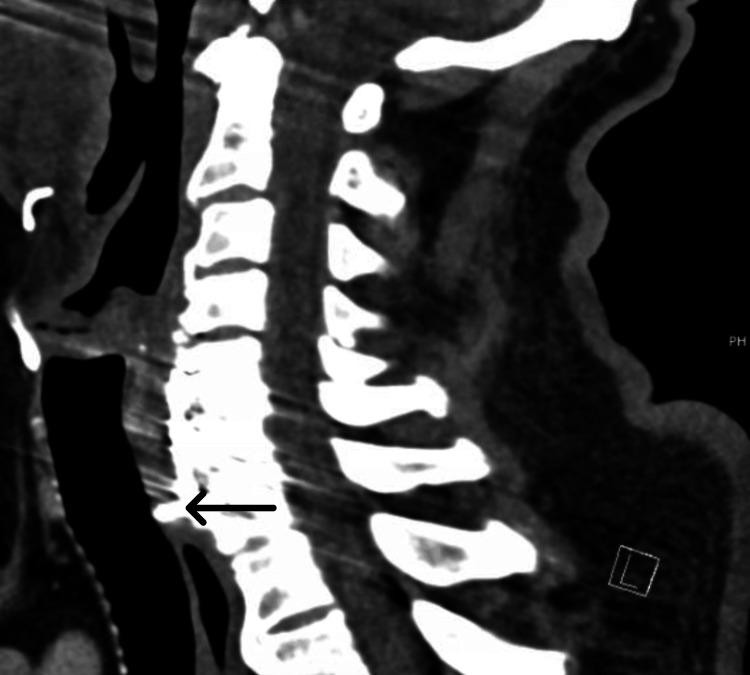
Computed tomography scan of the cervical spine with IV contrast reveals a C5-C7 anterior spinal fusion with a C7 screw protruding into the esophagus.
